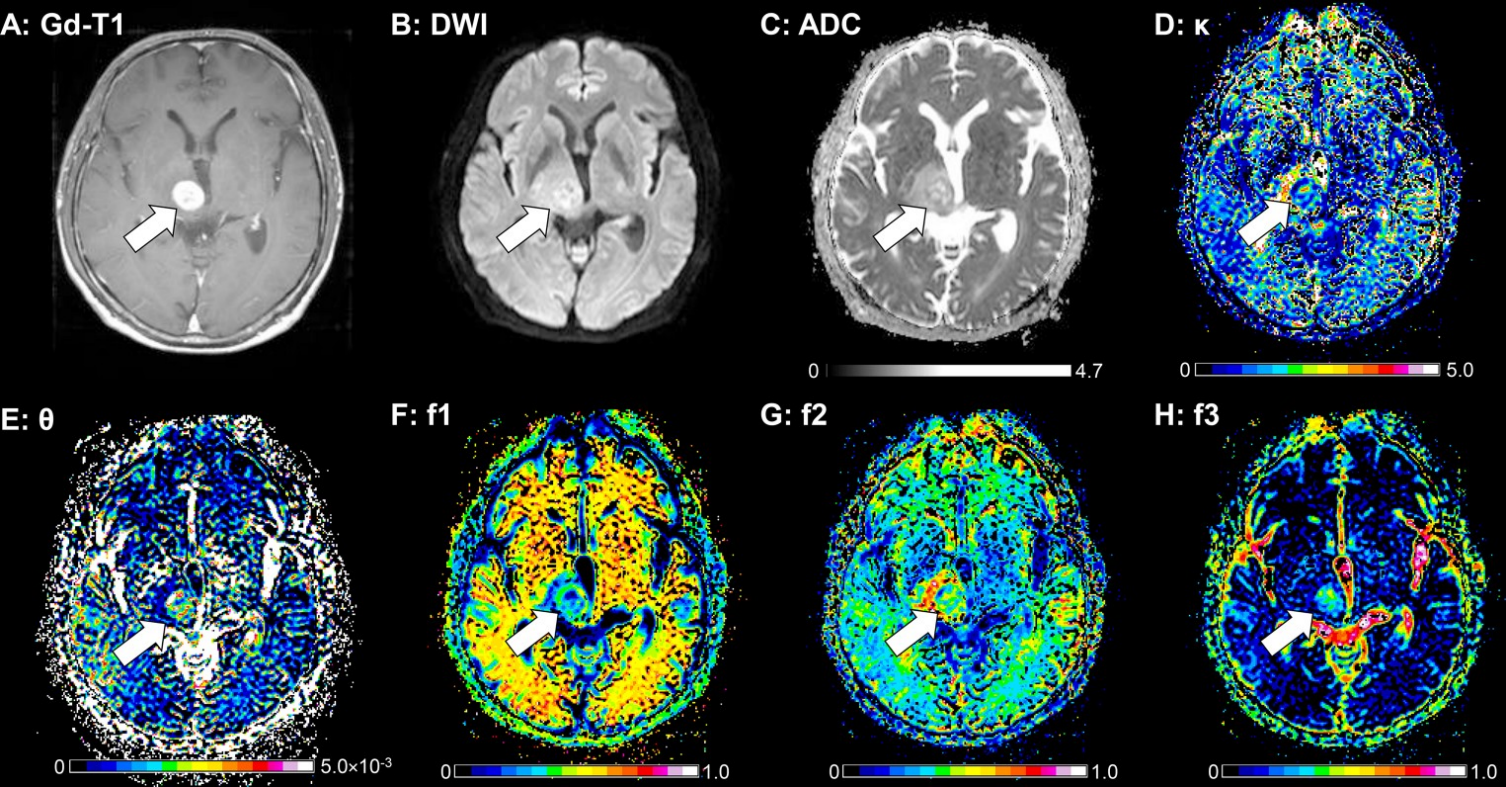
A 66-year-old-male with a GB. A: The post-contrast T1-weighted image shows a solid
